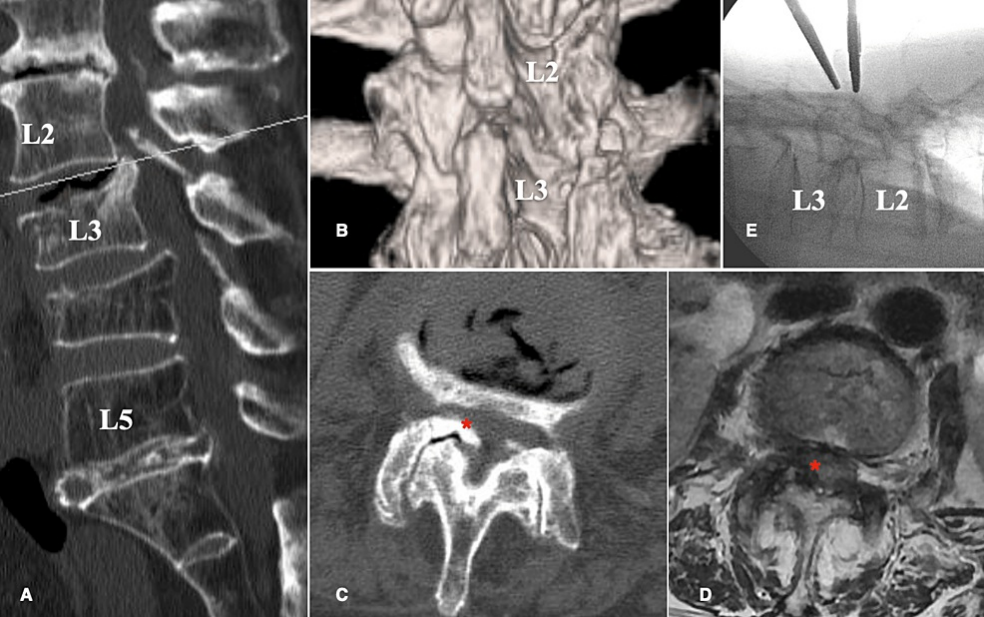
Preoperative imaging. (A) Sagittal CT confirmed an L3 compression fracture, and osteophytes protruded into the spinal canal. (B) Narrowing of the inter-laminar space was confirmed by 3DCT. (C) Axial CT showed osteophytes (*) extending from the superior articular process. (D) Axial MRI confirmed the compression of the dura mater (*). (E) The intraoperative lateral image was used to confirm that the working and camera portals form a triangle centered on the decompression site. 3DCT: 3-dimensional computer tomography.
